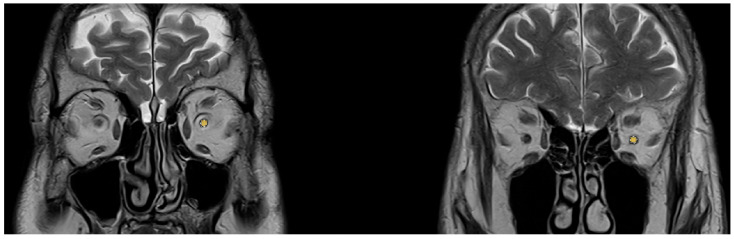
Coronal T2-weighted orbital MRI images used for optic nerve evaluation. The left panel represents a healthy control subject, while the right panel shows a patient with idiopathic Parkinson’s disease. ROI, shown in yellow, was placed on the intraorbital segment of the optic nerve for quantitative texture analysis.
